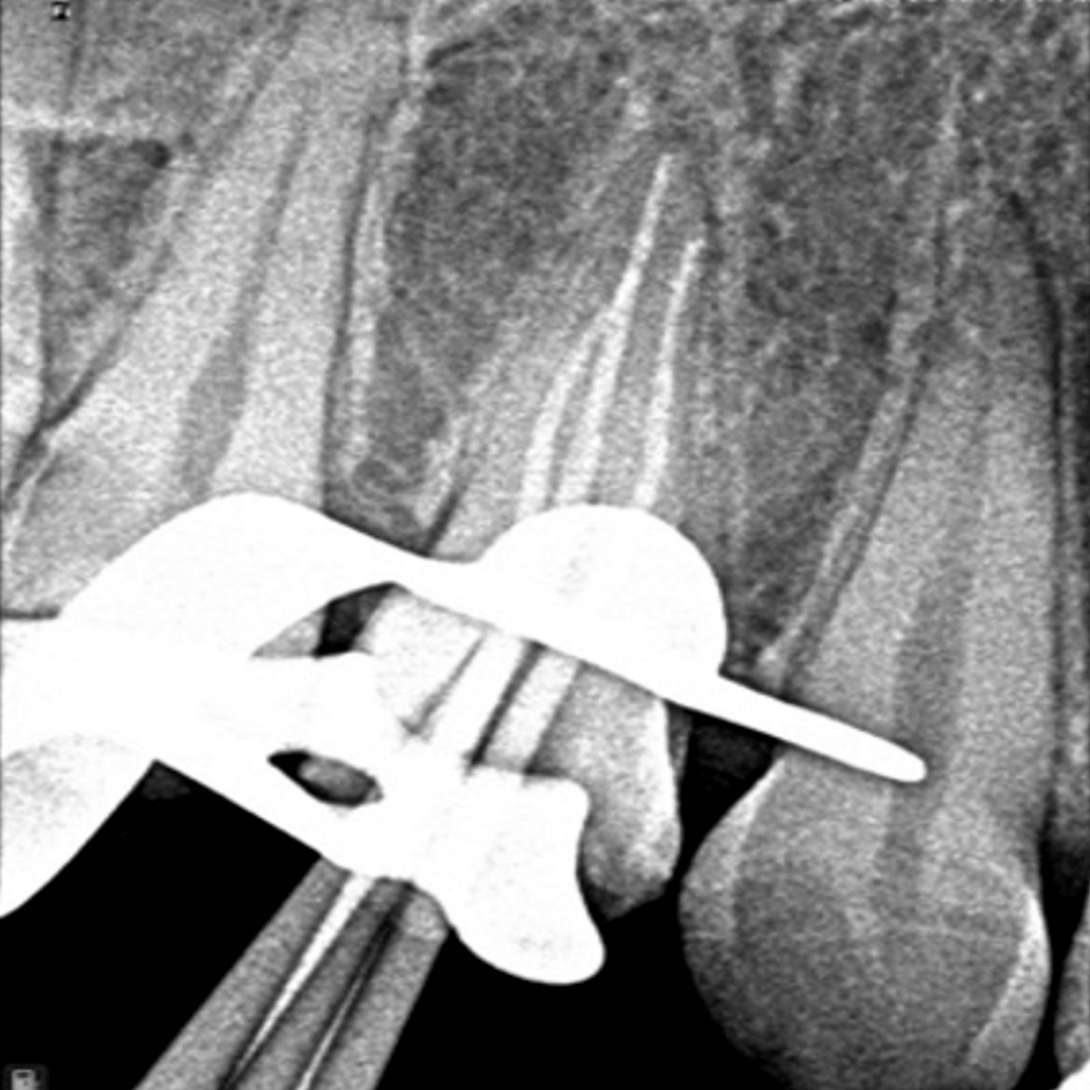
Radiograph of master cone selection
